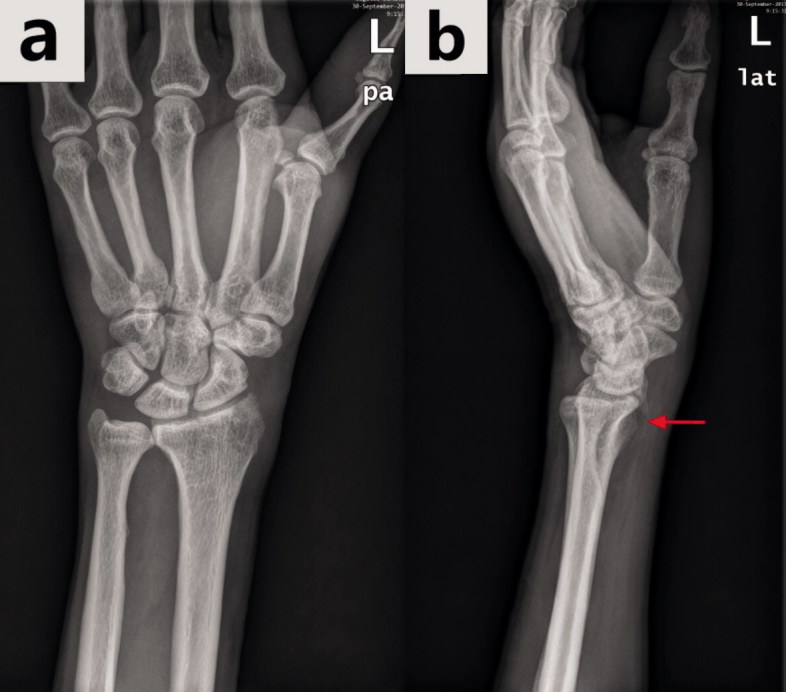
The same wrist with a DRF in the anterior–posterior view radiograph (a) and in the lateral view radiograph (b). The hidden DRF in the anterior–posterior view was apparent in the lateral view (the fracture is shown by the red arrow).
